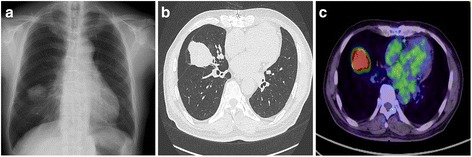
Radiological findings. a Chest X-ray shows an abnormal shadow of the right lung. b Chest CT shows a mass lesion with a smooth margin in the right middle lobe of the lung. c FDG-PET shows that the maximal standardized uptake value is 10.2 in the tumor
